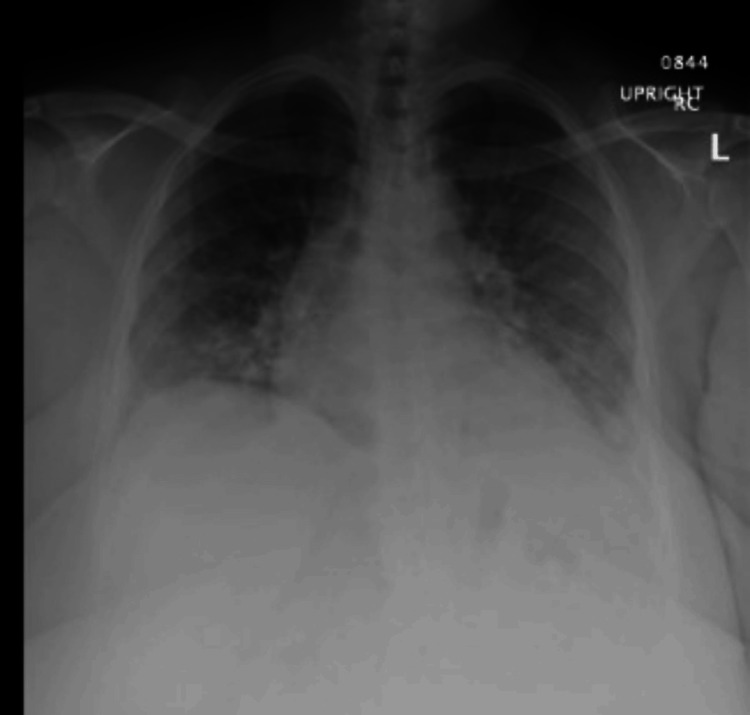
A chest X-ray obtained upon admission revealed emerging bilateral perihilar infrahilar patchy infiltrates, predominantly airspace in nature, extending inferiorly
